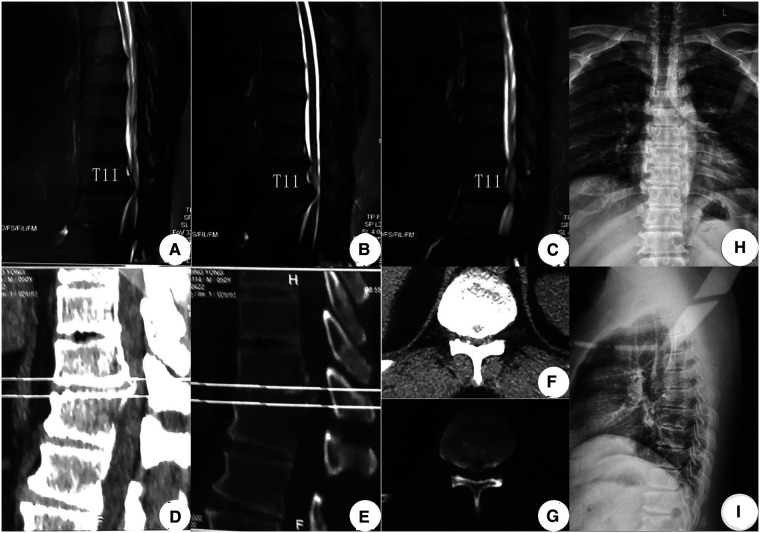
Preoperative image examinations (A–G). MR examination done on October 25, 2019, showed a giant TDH at the T11–12 levels: (A) Right sagittal T2WI, (B) middle sagittal T2WI, and (C) left sagittal T2WI. (D–G) CT examination recorded on November 14, 2019, confirmed CCTDH at T11/12: sagittal CT images [(D) soft tissue window; (E) bone window]. Transverse CT images [(F) soft tissue window; (G) bone window]. (H,I) Digital radiograph obtained on November 20, 2019, showed a normal thoracic sequence with no scoliosis. TDH, thoracic disc herniation; T2WI, T2-weighted image; CCTDH, central calcified TDH.
